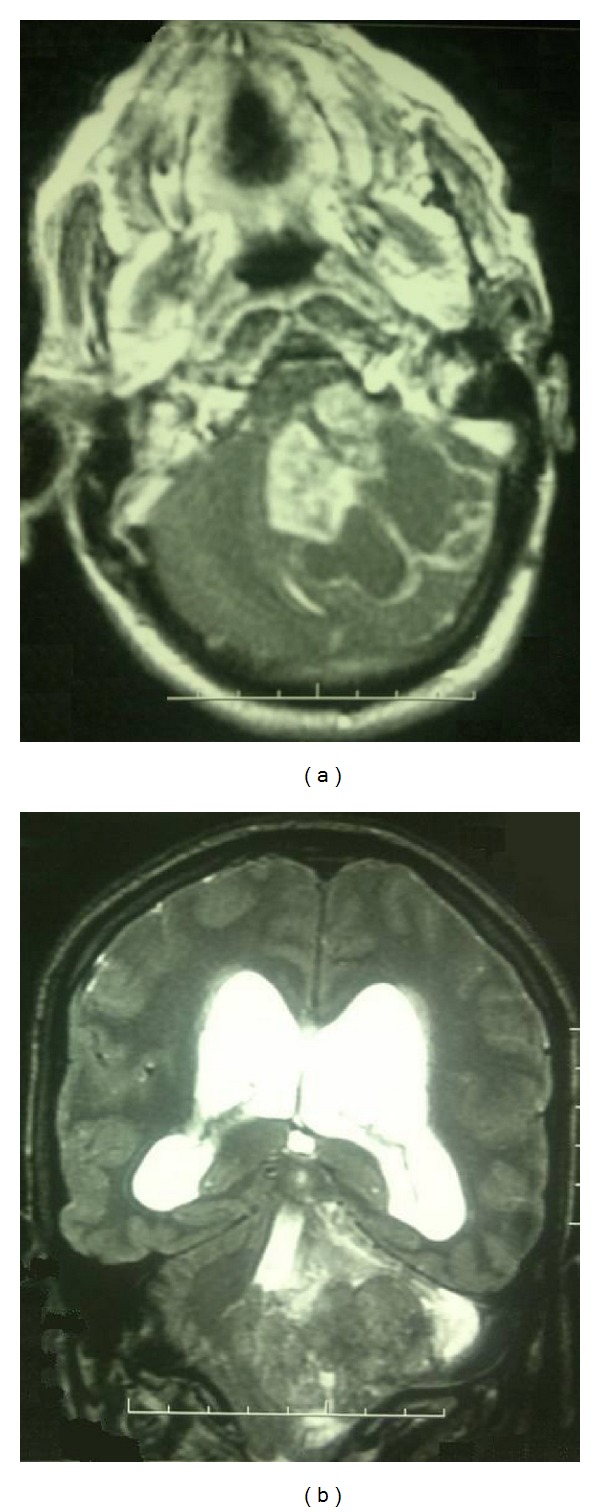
Axial (a) T1-weighted MRI with gadolinium and coronal; (b) T2-weighted MRI after the first surgery showing growth of the heterogeneous lesion with predominant enhancement and invading the surrounding structures.
